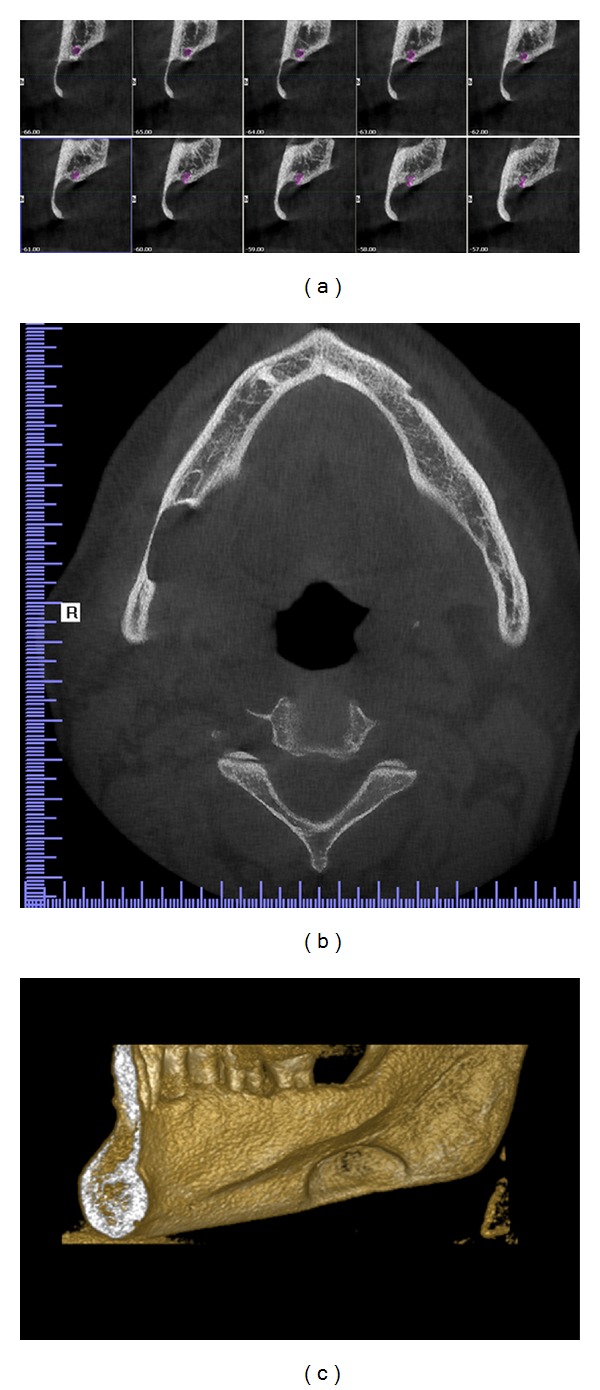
CBCT images: (a) sagital view displaying continuous 1 mm width sections, (b) horizontal view displaying the cavity outline, and (c) 3D reconstruction of the right mandible displaying buccal cortical bone reduction.
